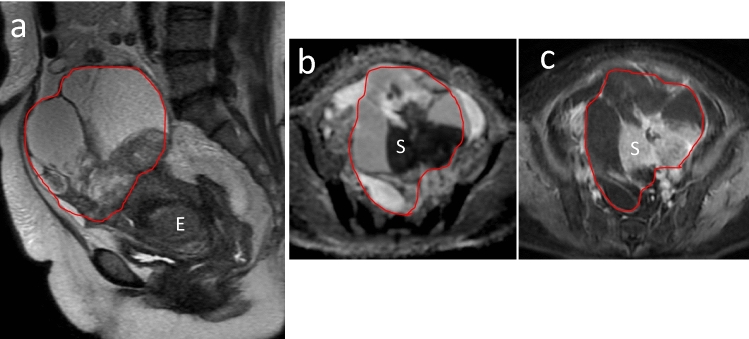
The red volume of interest manually drawn along the margin of the whole tumor in a 56-year-old woman with endometrioid carcinoma. a Single-shot fast-spin echo T2-weighted image showing solid and cystic (one-third to two-thirds solid component) tumor. Endometrial carcinoma (E) revealed thickening of the uterine endometrium. b Apparent diffusion coefficient map showing hypo-intensity in the solid component (S) within the tumor, indicating restricted diffusion referring to the myometrium. c Contrast-enhanced image on equilibrium phase showing hypo-intensity in the solid component (S) referring to the myometrium
